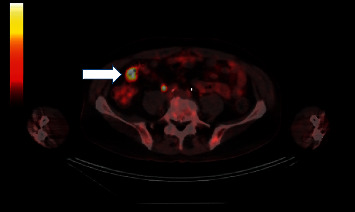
PET (positron emission tomography) scan with persistent and increasing FDG uptake in distal small bowel (arrow).
